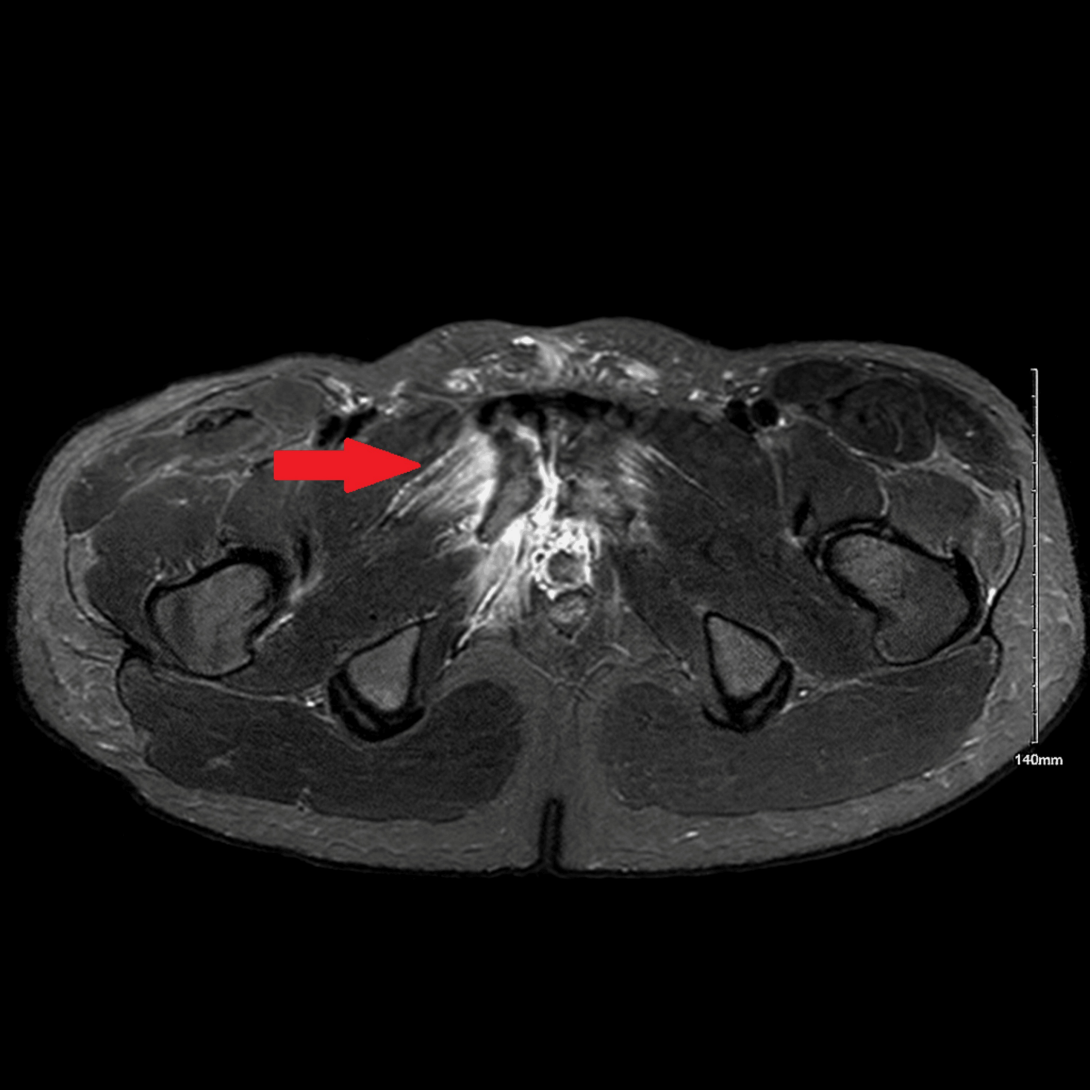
MRI axial view The arrow denotes edema and foci of fluid in the vicinity of the pubic symphysis and the right obturator internus muscle.
